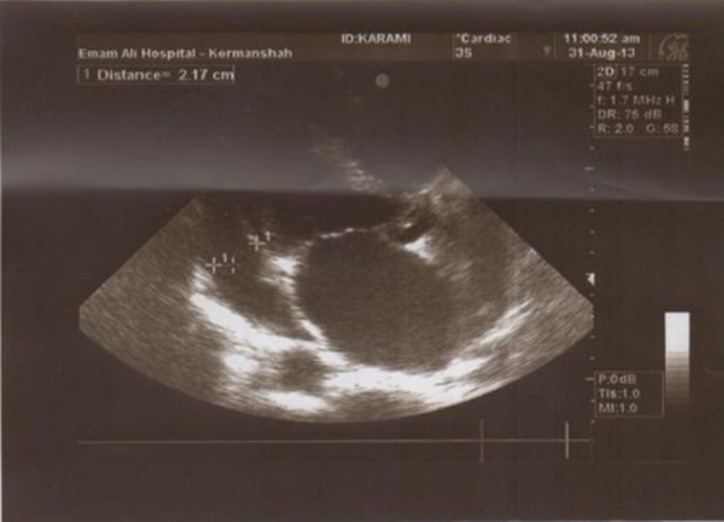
Echocardiography in four chamber view shows pericardial effusion
